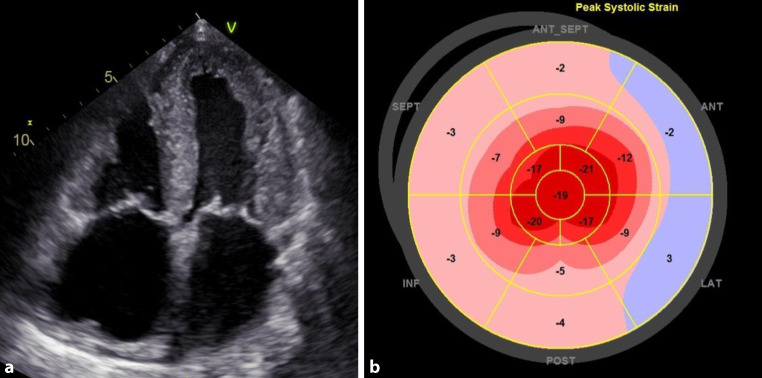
Echocardiographic findings in cardiac transthyretin amyloidosis. a Apical four-chamber view showing pronounced thickness of the left and right ventricular walls and the interatrial septum, enlargement of both atria and slight pericardial effusion. b Global longitudinal strain analysis in cardiac transthyretin amyloidosis showing apical sparing as a typical sign (i.e., cherry on the top phenomenon). *SEPT* septal, *ANT* anterior, *INF* inferior, *ANT_SEPT* anterior septal, *LAT* lateral, *POST* posterior
